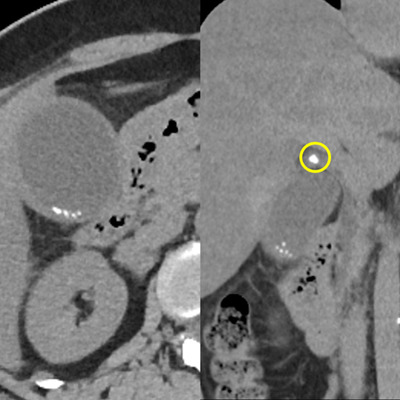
Abdominal computed tomography 4 days after admission. Swollen gallbladder with gallstones and wall thickness. The yellow circle in the coronal section indicates an impacted cystic duct stone
